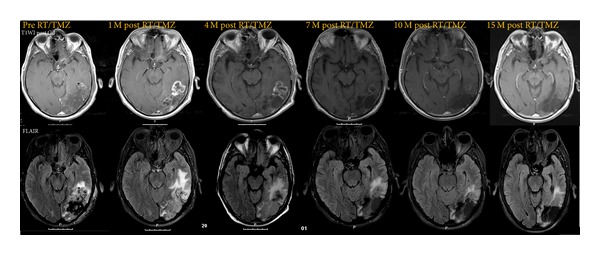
T1-contrast-enhanced and FLAIR magnetic resonance imaging (MRI) documenting pseudoprogression. Compared with pre-RT/TMZ MRI, the 1-month post-RT/TMZ showed increasing areas of contrast enhancement suggestive of tumoral progression. The patient remained clinically stable with stable dose of corticotherapy. Adjuvant TMZ treatment cycles were maintained and serial MRI at 4, 7, 10, and 15 months showed a consistent reduction in the size and the contrast of the lesion as well as in FLAIR signal.
